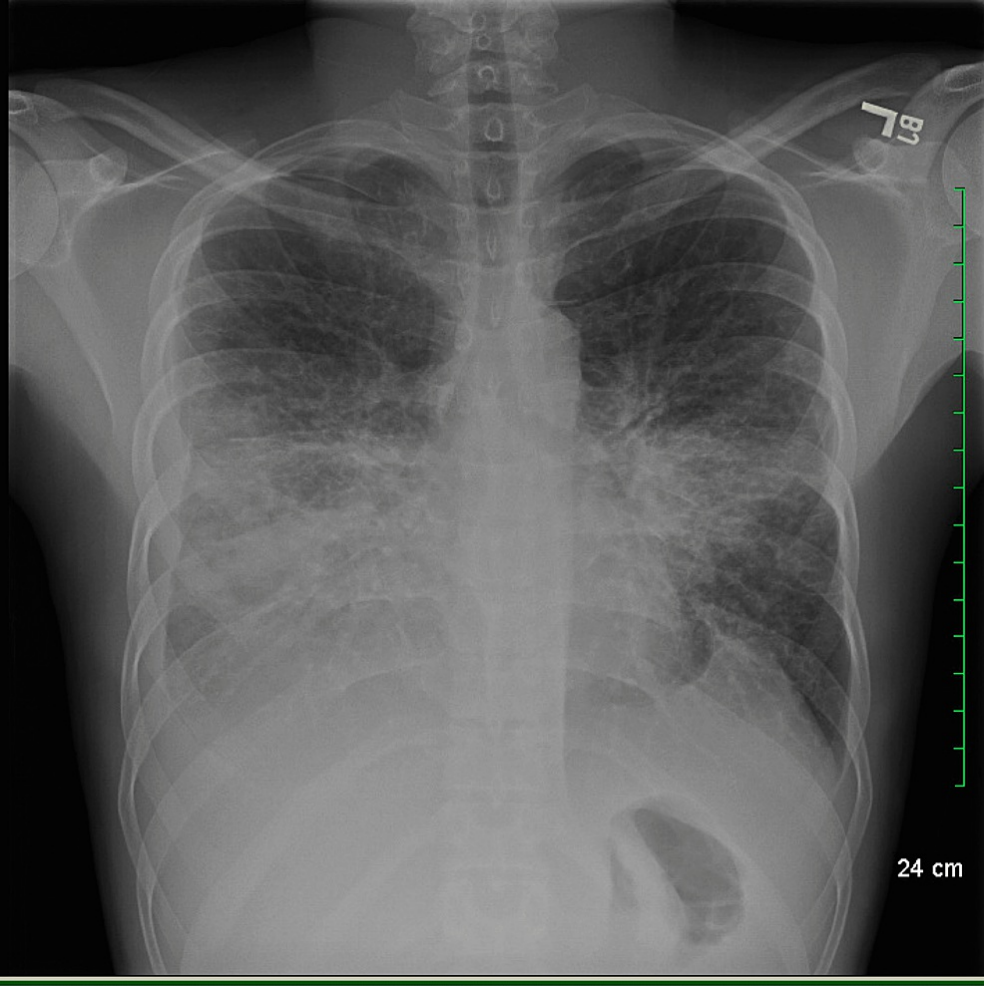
Patient's initial chest x-ray on admission.
